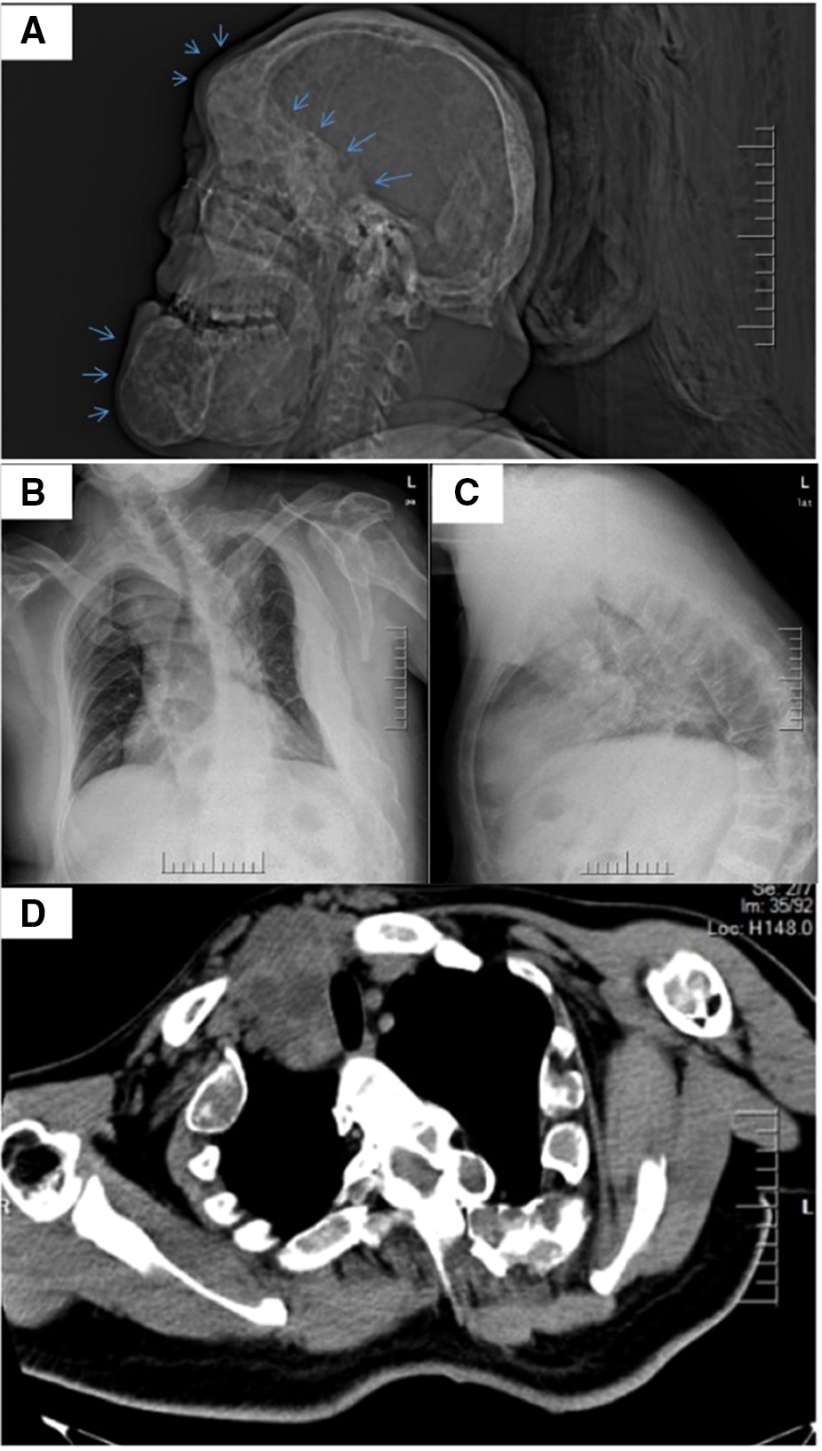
Radiographic features of the patient before surgery demonstrating fibrous dysplasia and an enlarged thyroid. The lateral skull radiograph acquired from a CT (A) indicates polyostotic fibrous dysplasia on the head (blue arrows). X-ray from the patient (B,C) shows thoraco-lumbar scoliosis. CT image of patient upper chest (D) demonstrates the enlarged retrosternal goiter.
